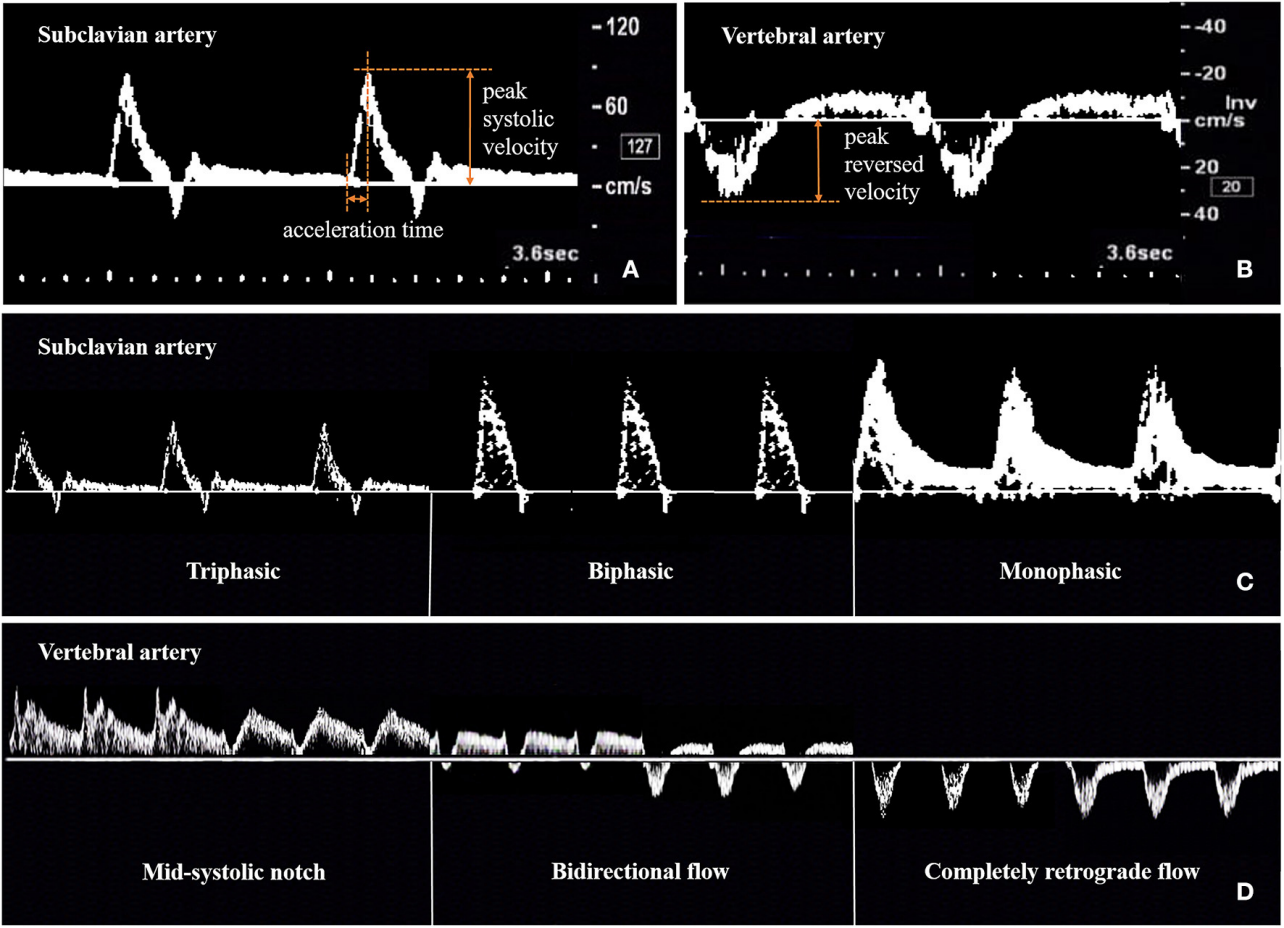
Method for measuring and recording ultrasonic parameters of subclavian artery (A,C) and vertebral artery (B,D).
